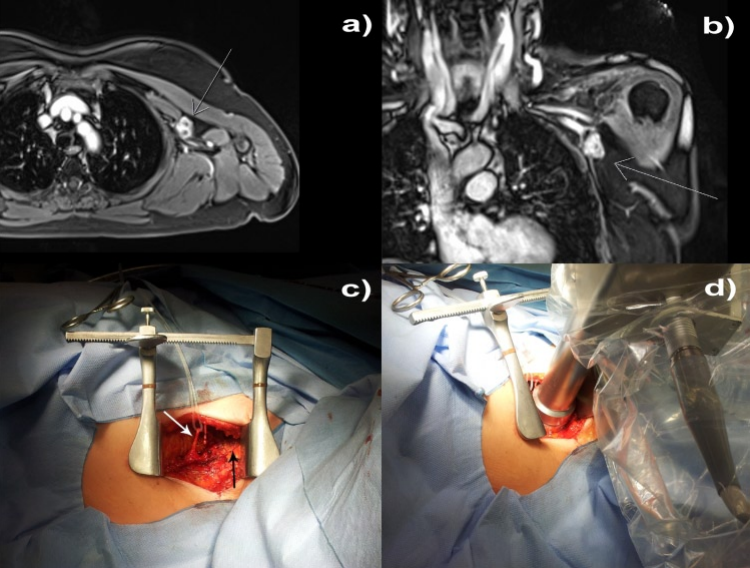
Intraoperative treatment: (a) axial and (b) coronal magnetic resonance images showing the relationship between the 3 cm irregular auxiliary mass to the auxiliary vessel/nerve complex; (c) the auxiliary vessels exposed (black arrow), thoracodorsal nerve placed aside (white arrow); and (d) the applicator setup.
